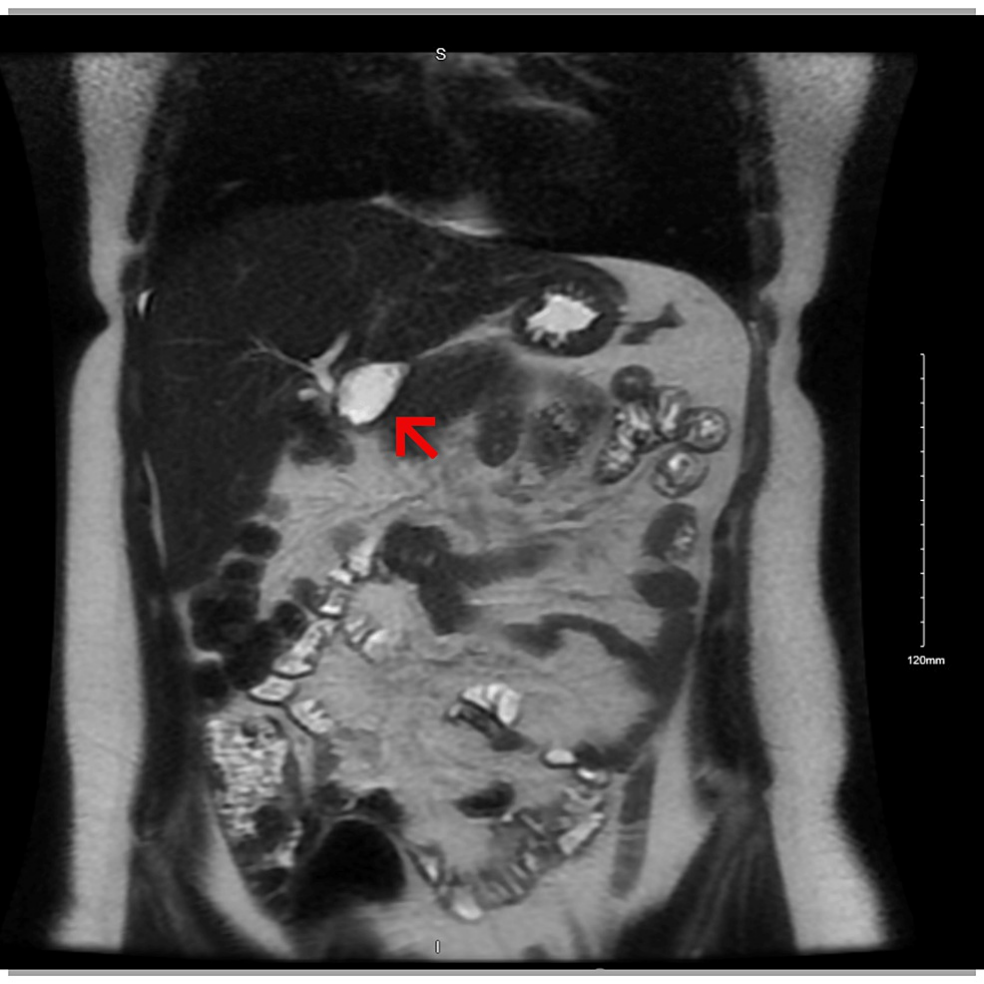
Coronal view of abdominal MRI with contrast. The structure is considered as a possible left-sided gallbladder (arrow), but as seen in Figure 5, this is more likely consistent with the post-pyloric duodenum.
